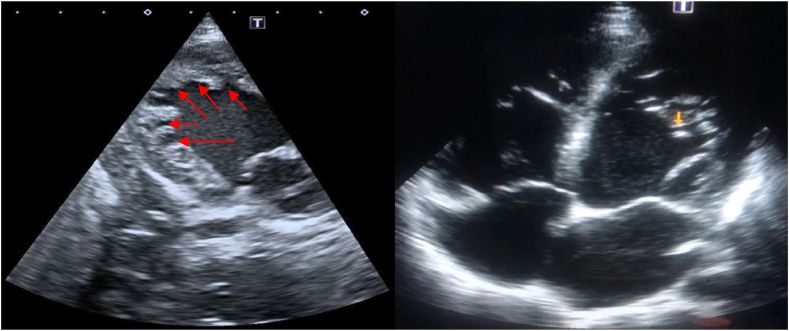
Panel A Para-sternal short axis views. Panel B apical 4 champers with left ventricular shows trabiculaion.
